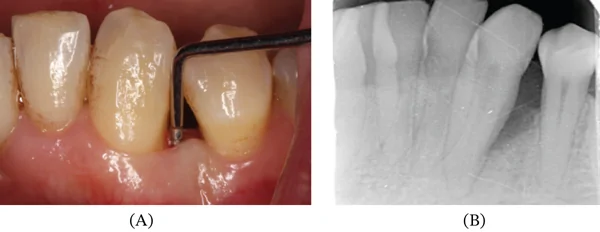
(A) PD measurement 9 mm and (B) radiographic evaluation.
